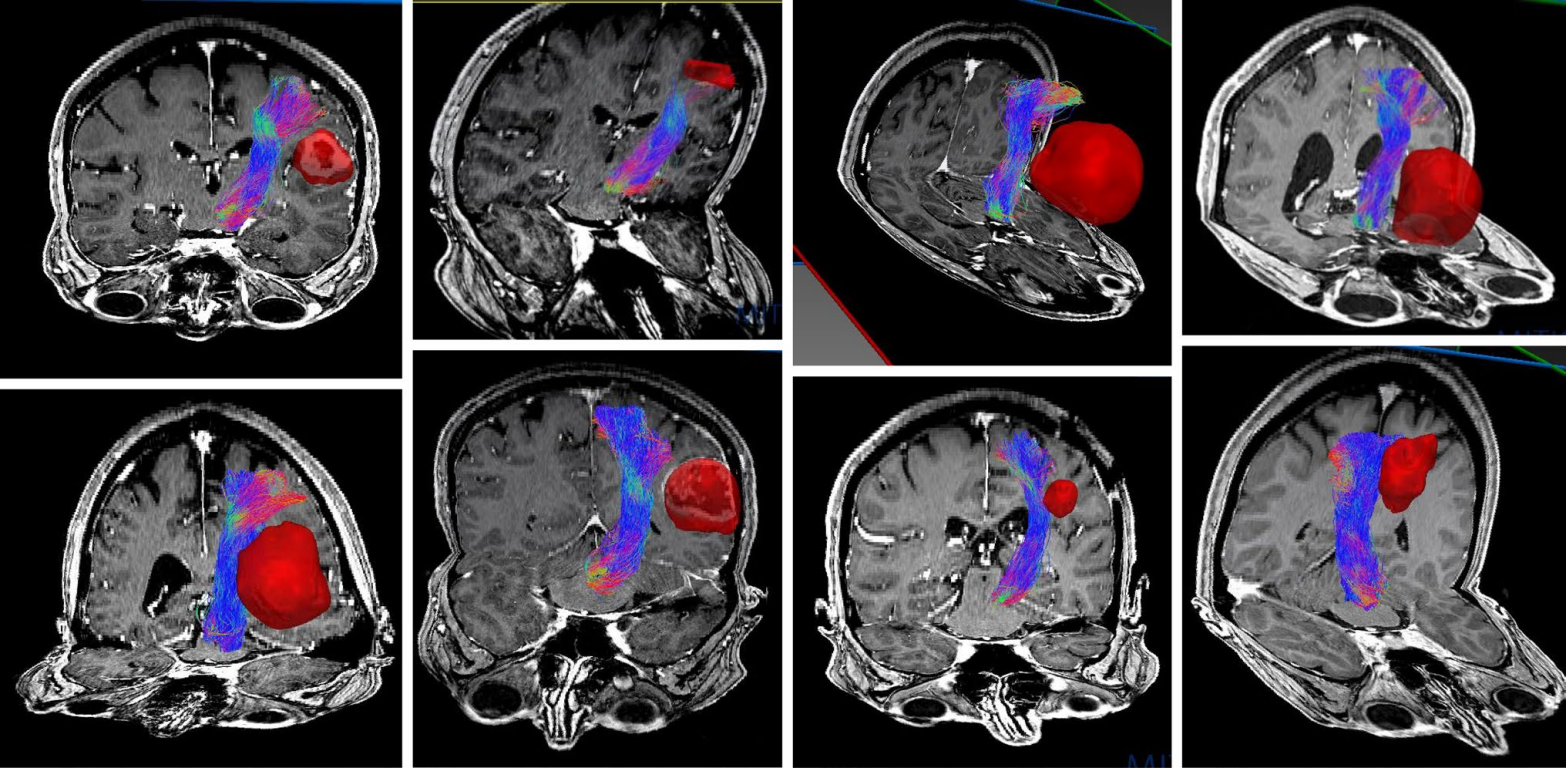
Overview of CST FT results. Tumors displayed in red. MRI T1-weighted, 3D view, cropped. All Rater 1, QBI-FT. (CST, corticospinal tract; QBI, Q-ball Imaging; FT, fiber tractography)
